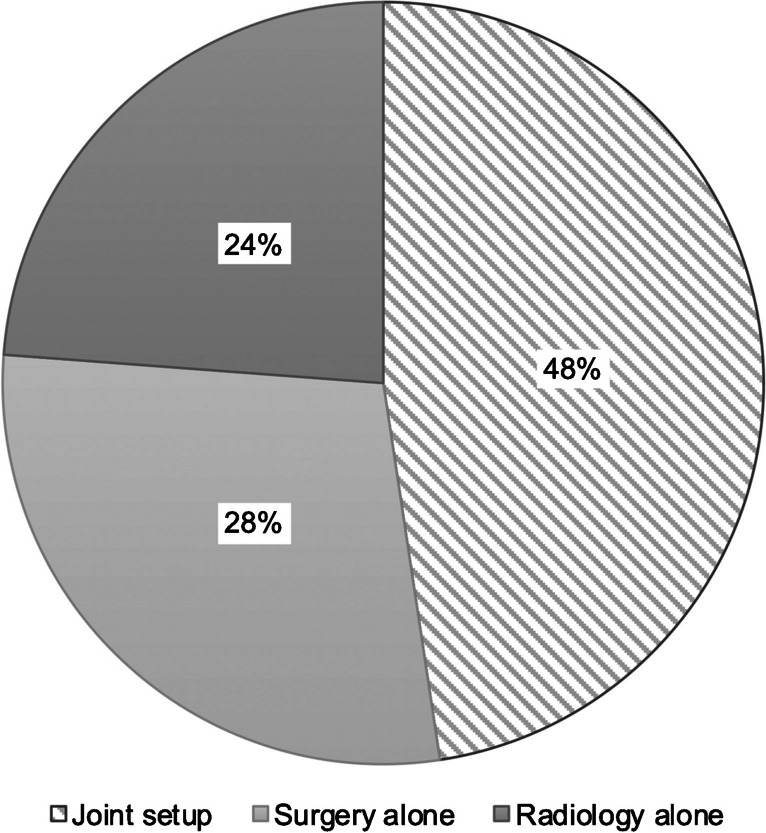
Usual setup for liver transplantation intraoperative ultrasound
